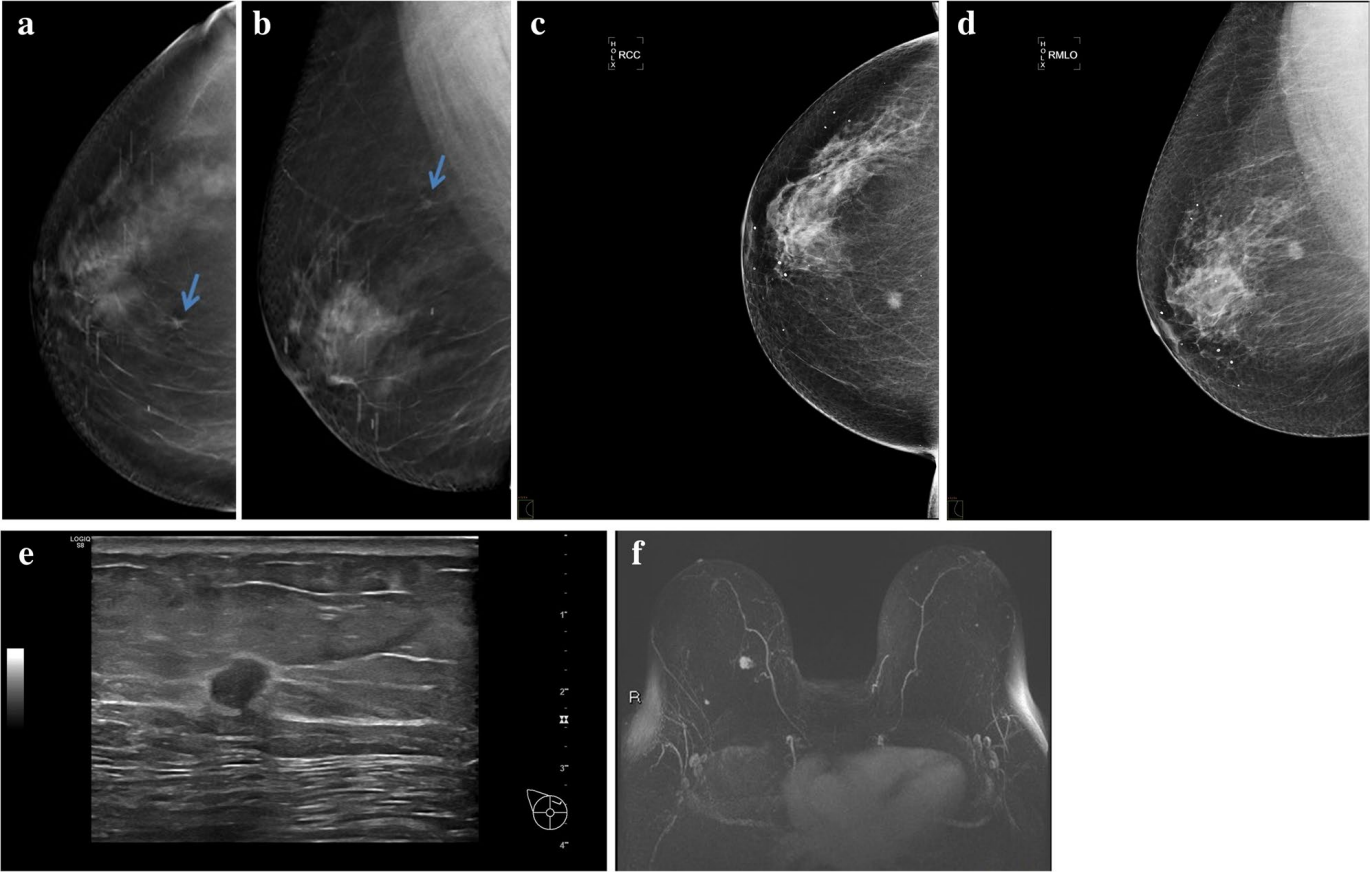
DBT interval carcinoma. A 60-year-old asymptomatic patient who underwent a repeat mammographic study 20 months after the screening study interpreted as normal due to the diagnosis of breast cancer in a first-degree relative. Craniocaudal (a) and mediolateral oblique (b) projections, respectively, of the tomosynthesis of the right breast performed in the screening program, in which a small spiculated mass is identified in the upper inner quadrant of the right breast (arrow) that was not noticed at the time. Craniocaudal (c) and mediolateral oblique (d) projections, respectively, of the diagnostic mammogram showing that the mass had increased in size. e Breast ultrasound, in which the mass is visible. f Breast MRI. MIP T1 reconstruction with IV contrast and fat suppression in which it is verified that the lesion is unique (arrow). Pathological result: luminal A grade 2 invasive ductal carcinoma
